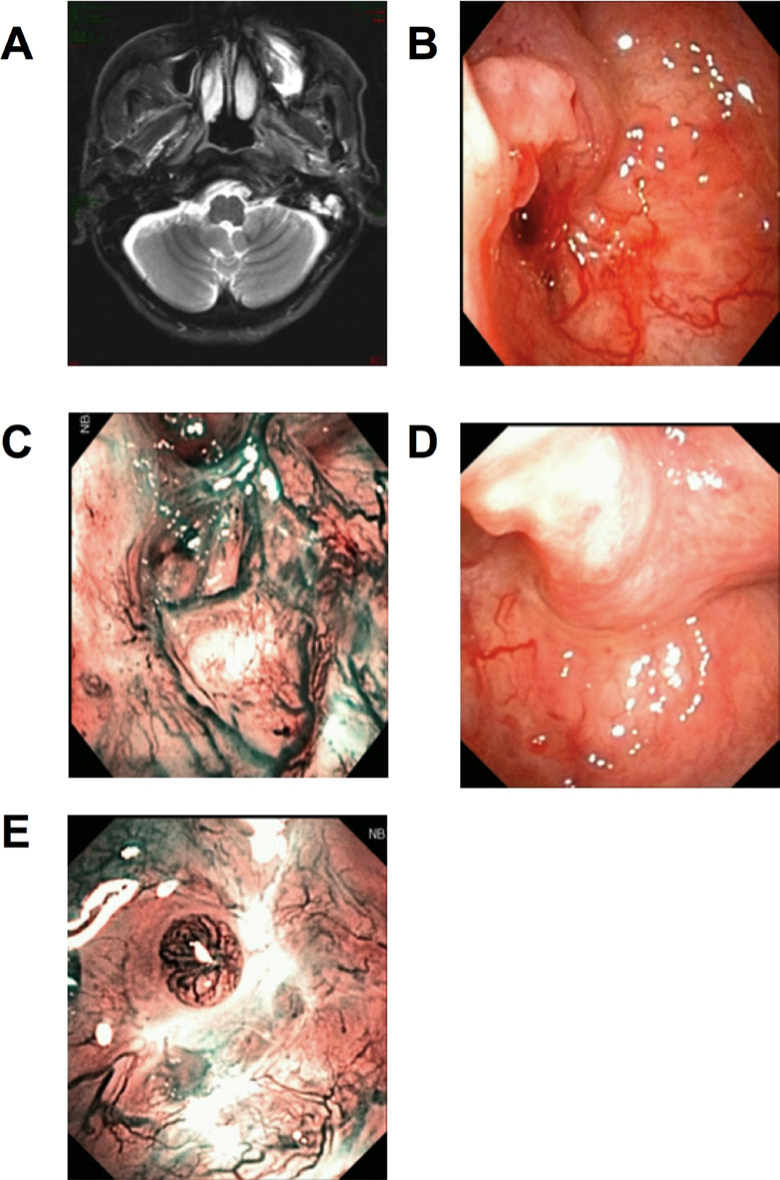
Patients with first diagnosed NPC Head and Neck MRI (T2WI + contrast) scan showed the slightly blunt fossa of Rosenmuller, local bulging mucosa and slightly high signal swollen medial pterygoid and longus capitis on the left side (A). Under WL, part of the torus tubarius was missing by forceps biopsy (B). Under NBI, mucosa in biopsy area was hyperemia, there was no positive found as brownish spots (C). The CNB puncture point with distance and closer view 2 weeks later, under WL and NBI respectively (D and E).
